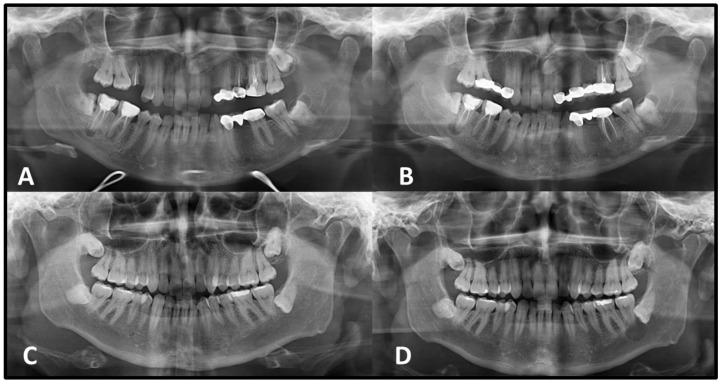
A unilocular radiolucent lesion with well-defined corticated margins associated with an impacted left mandibular third molar is seen on the initial panoramic radiograph (A). The lesion appeared radiographically stable with no significant changes two years later (B). A radicular cyst with well-defined corticated margins associated with an impacted left mandibular third molar is seen on the initial panoramic radiograph (C). After a 5-year interval, the lesion exhibited mild enlargement and extended into the inferior alveolar canal (D).
